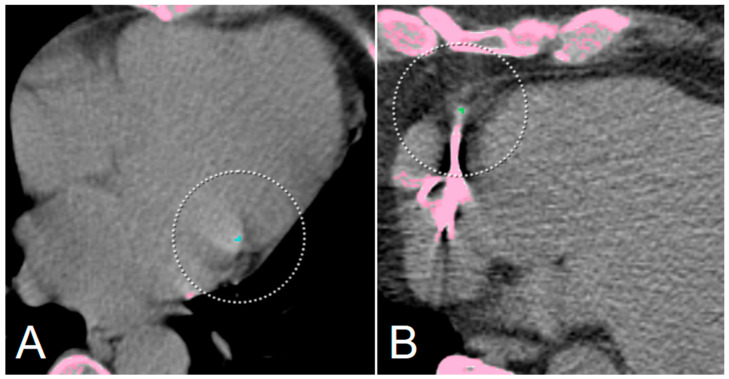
Two representative false-positive ratings from the AI-CACS tool on ungated CT from PET/CT. No coronary calcifications are present in a 66-year-old woman undergoing PET/CT for staging of gastric cancer (A), however, small calcifications of mitral valve were falsely marked as ramus circumflexus CAC. In another 66-year-old male patient (B), no CAC was found in dedicated gated CAC scan, however, small areas of increased density due to image noise adjacent to a pacemaker electrode were marked as calcification. Note: Pink areas are highlighing dense areas in the CT image (i.e., bone or calcification), with the circles indicating coronary artery calcification.
